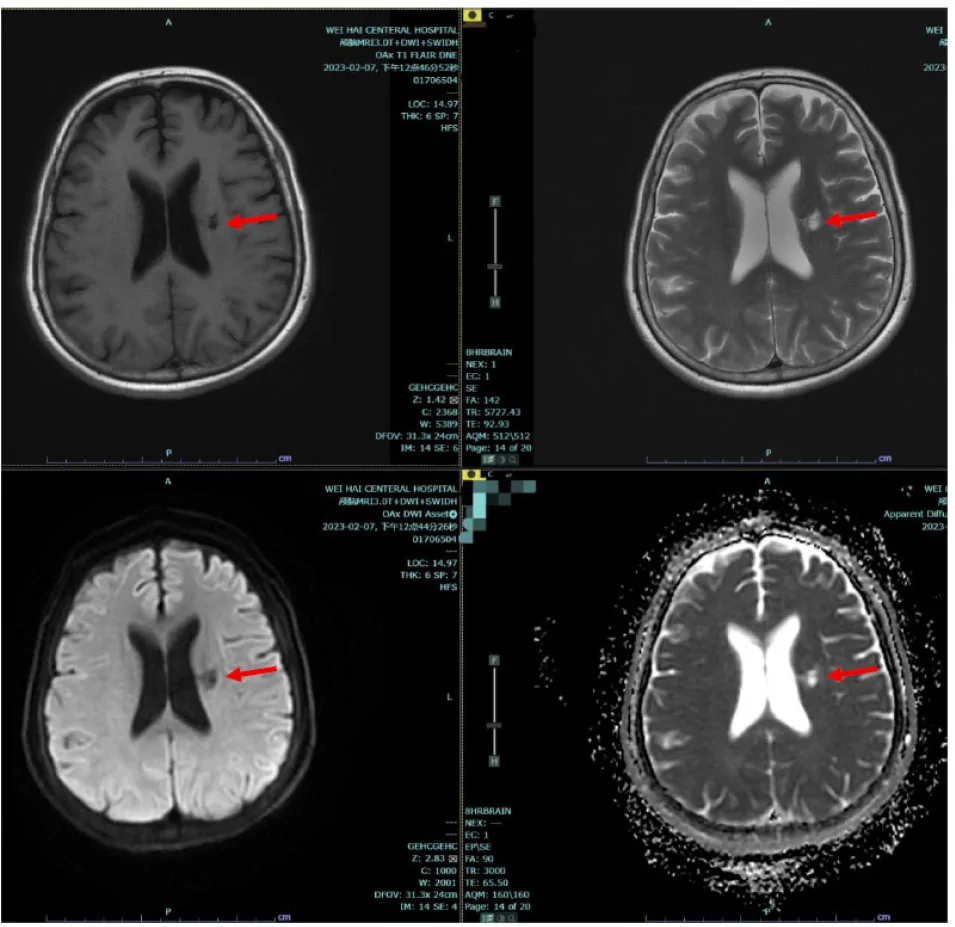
Brain MRI from February 7, 2023, shows patchy areas of prolonged T1 and T2 signal intensities in the left basal ganglia, with low signal on DWI and high signal on FLAIR.
